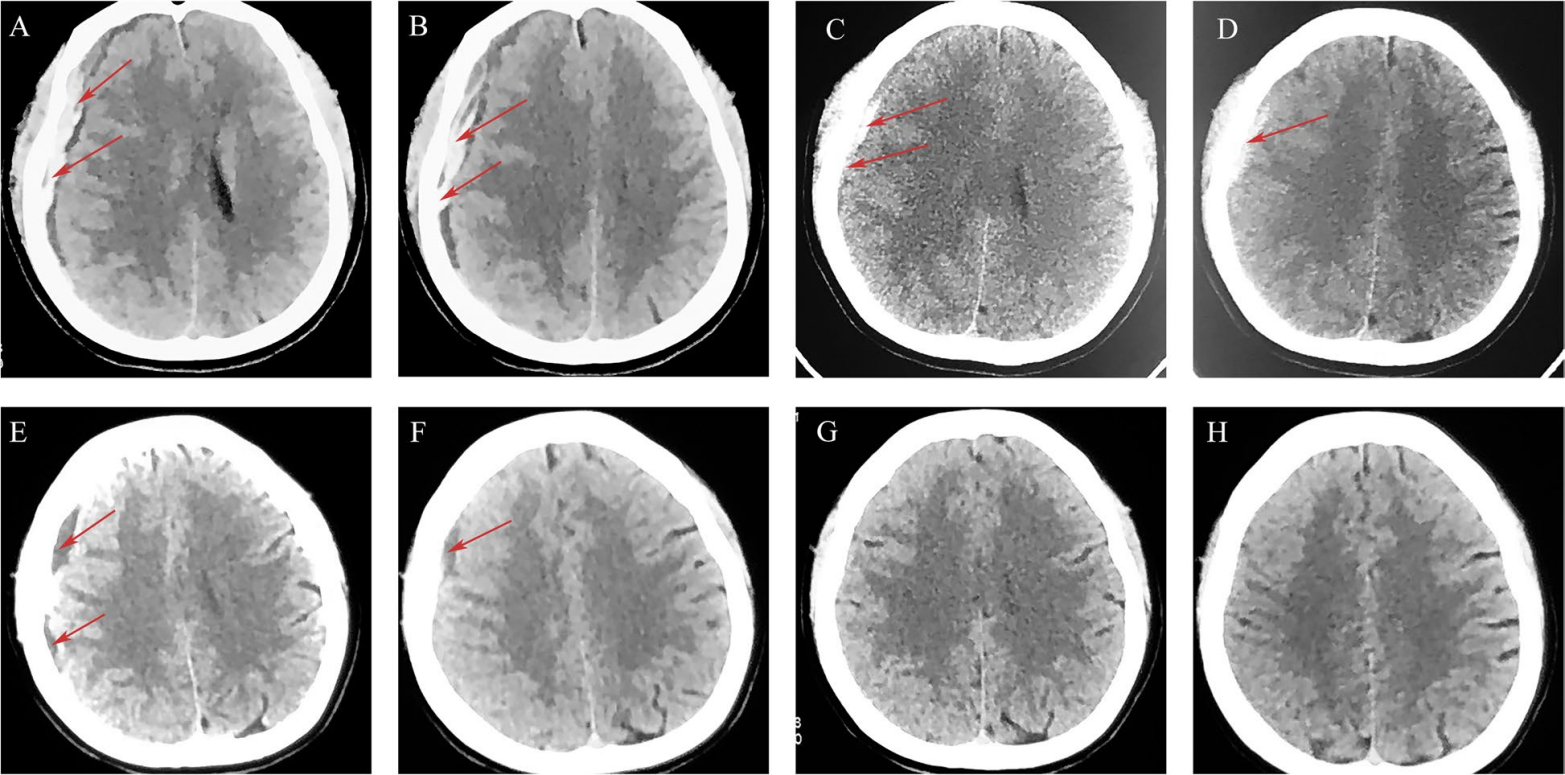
CT scan images for patient 1. ASDH (red arrows) was detected from initial brain CT (A, B). Follow-up CT scan on the 7th hospital day revealed that the hematoma was reduced (C, D). After 4 weeks of conservative treatment, the right-side hematoma becomes low-density hydrops (E, F). Complete resolution of the hematoma was observed after 2 months (G, H)
